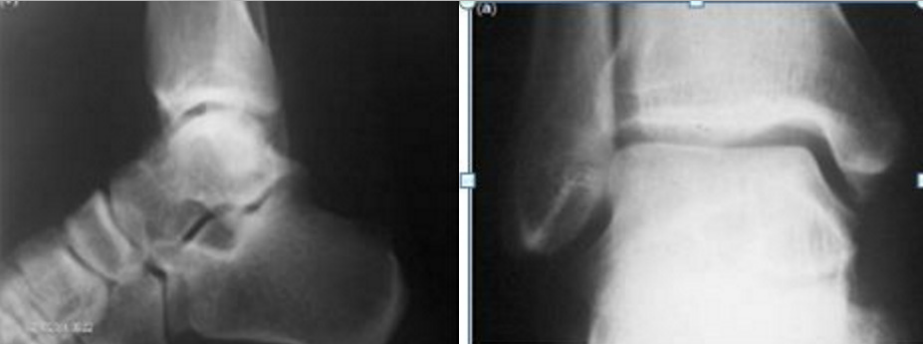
Radiographie de la cheville face et profil évidence une plage hétérogène en regard du col du talus
